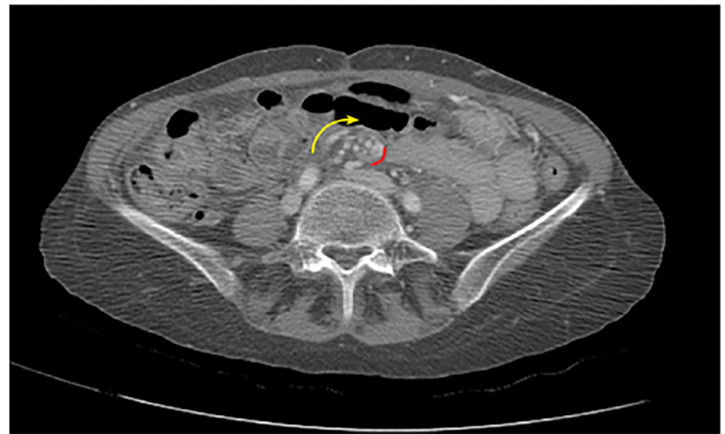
Still images: Sequential computed tomography images demonstrate small bowel and mesenteric vessels rotating in mass with soft tissue and fat attenuation. Hash mark highlights the position of a specific piece of mesentery as it revolves; arrow indicates movement of the mass.
